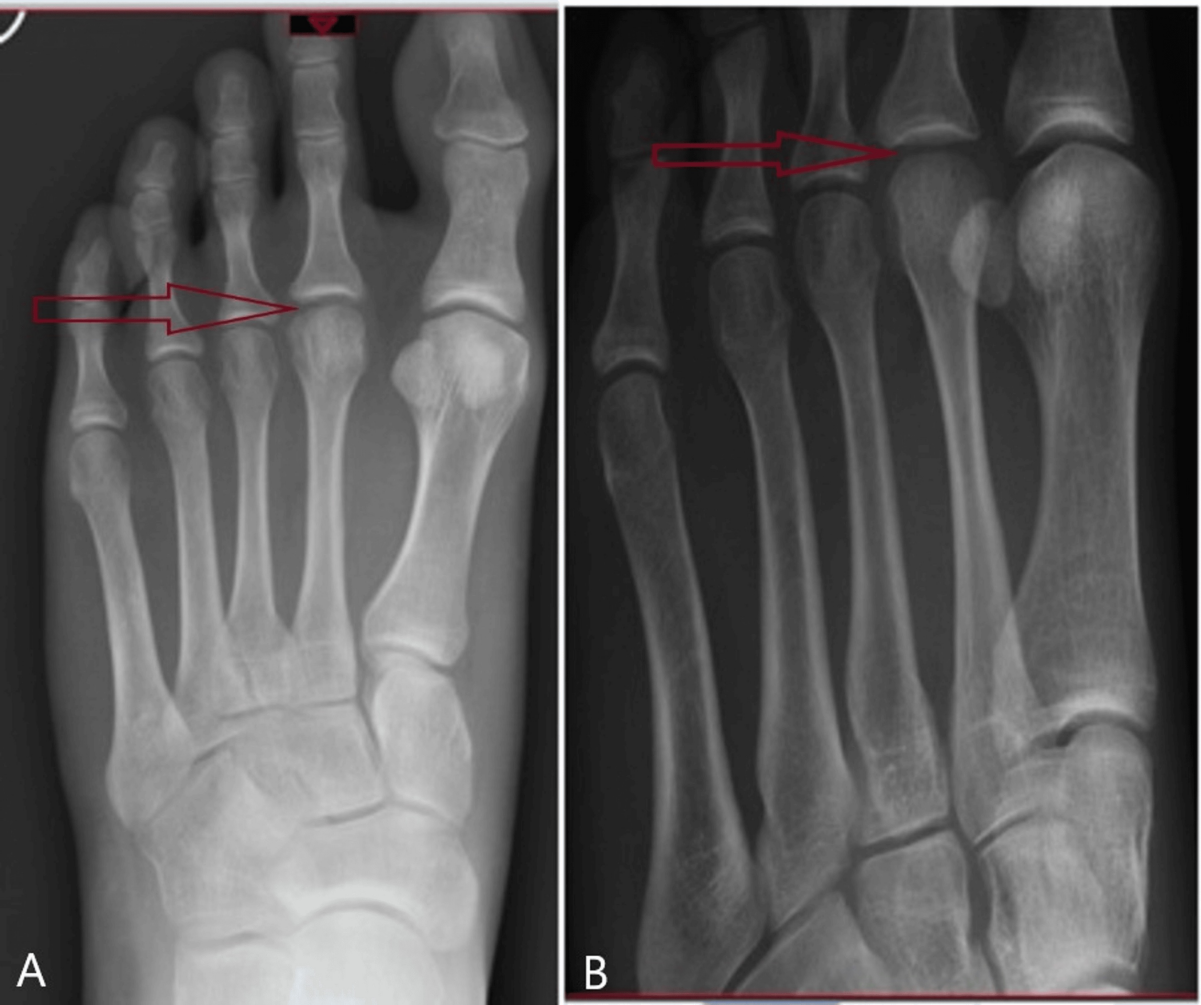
X-rays at the final follow-up showed complete remodeling of the second metatarsal head. A: AP X-ray at the final follow-up showing complete remodeling of the second metatarsal head B: Oblique X-ray at the final follow-up showing complete remodeling of the second metatarsal head
